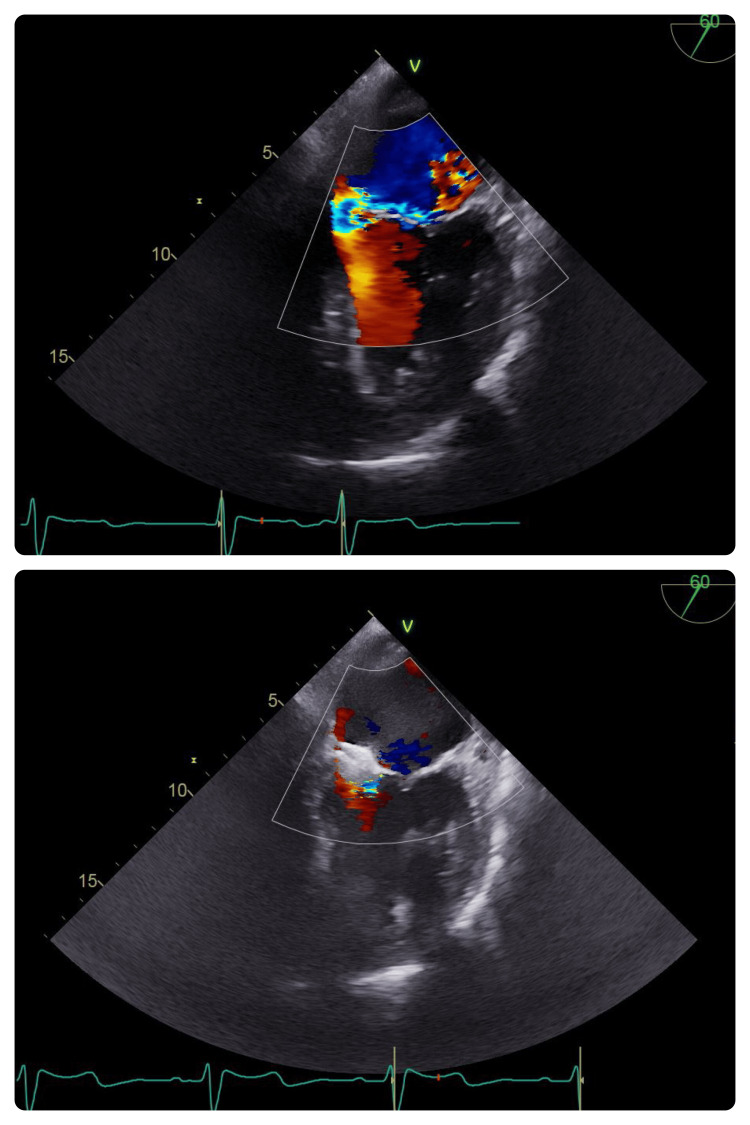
Transesophageal echocardiogram view at 60°. Top: a significant reduction in MR after deployment of the Amplatzer device. Bottom: stable deployment of the Amplatzer device MR: mitral regurgitation
